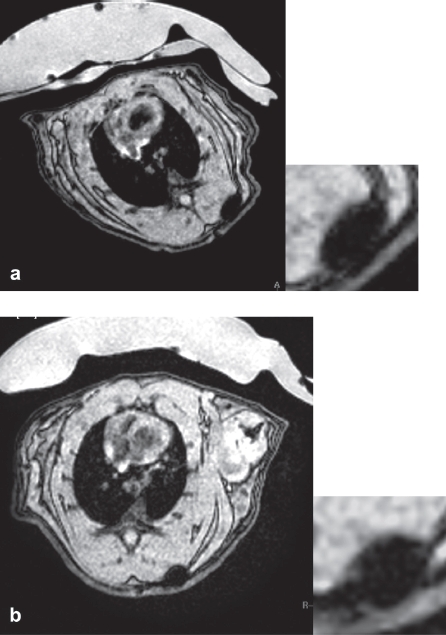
1 × 10^6^ MSC (passage 4) labeled with MU-Wuest 4 100 μg Fe/mL in 1 mL of a collagen scaffold 2 × 3 × 2-mm subcutaneous implant, (a) MRI taken 2 days after implantation; (b) MRI 25 days after implantation. As a control, the same number of unlabeled MSC in a scaffold was implanted on the contralateral side. No *T*_2_/*T*_2*_ signal could be detected at the implantation site of the control.
